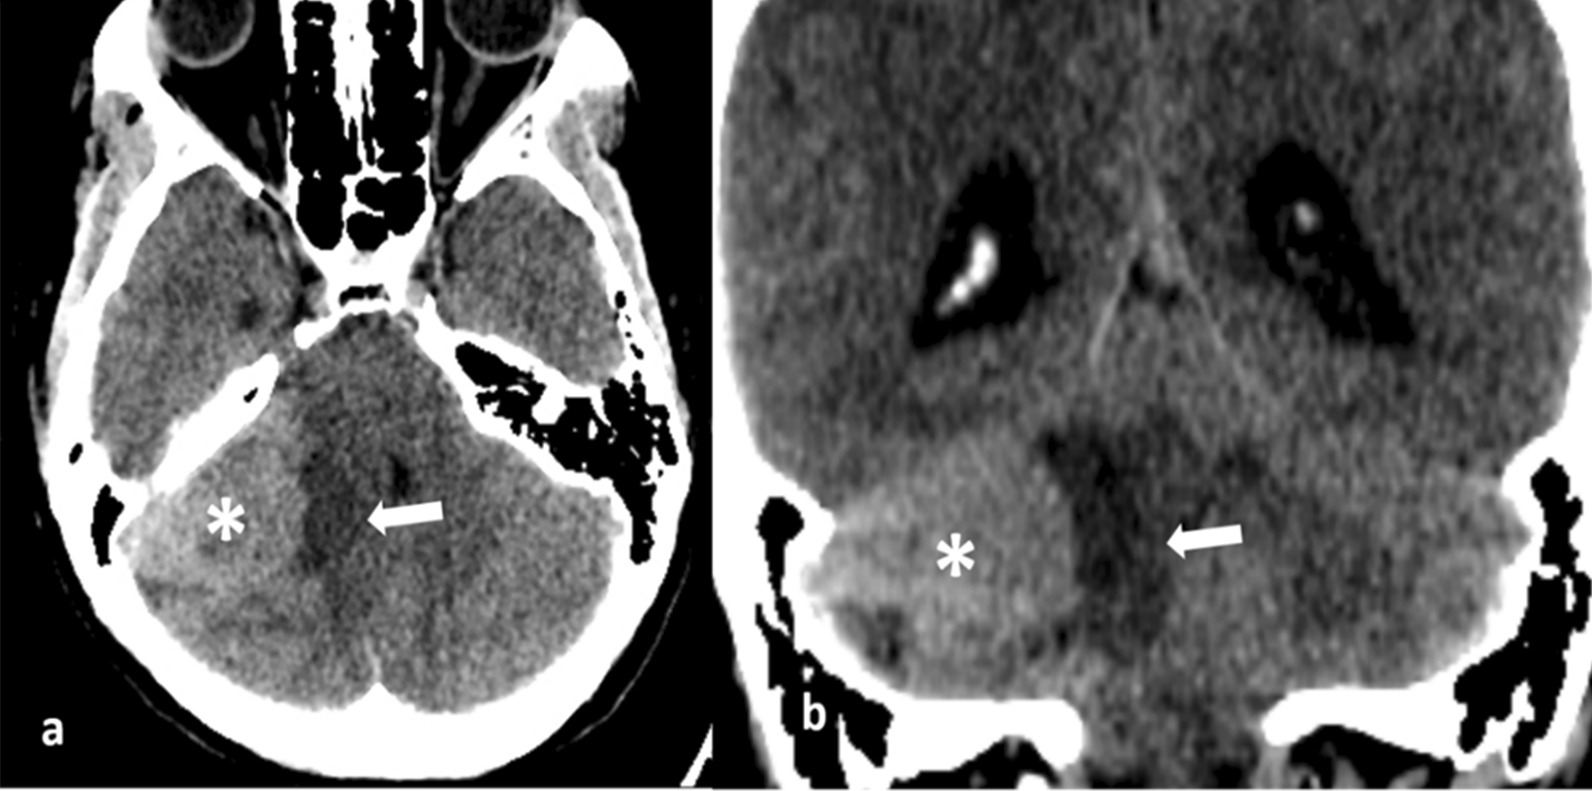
Nonenhanced computed tomography image on the axial plane (a) and coronal plane (b) showing a right cerebellopontine angle mass (asterisk) that is hyperdense compared with normal tissues. Moderate cerebral edema surrounding the mass can be observed (arrow)
